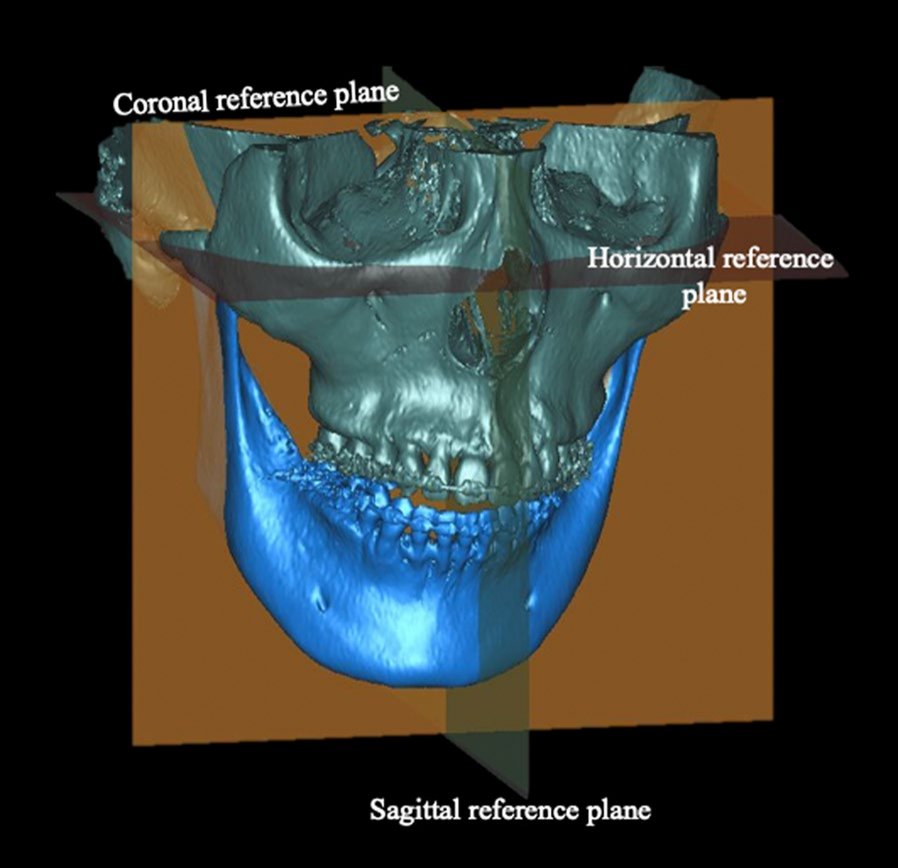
Three-dimensional computed tomography image of a patient with mandibular asymmetry with menton deviation greater than 4 mm from the mid-sagittal plane
